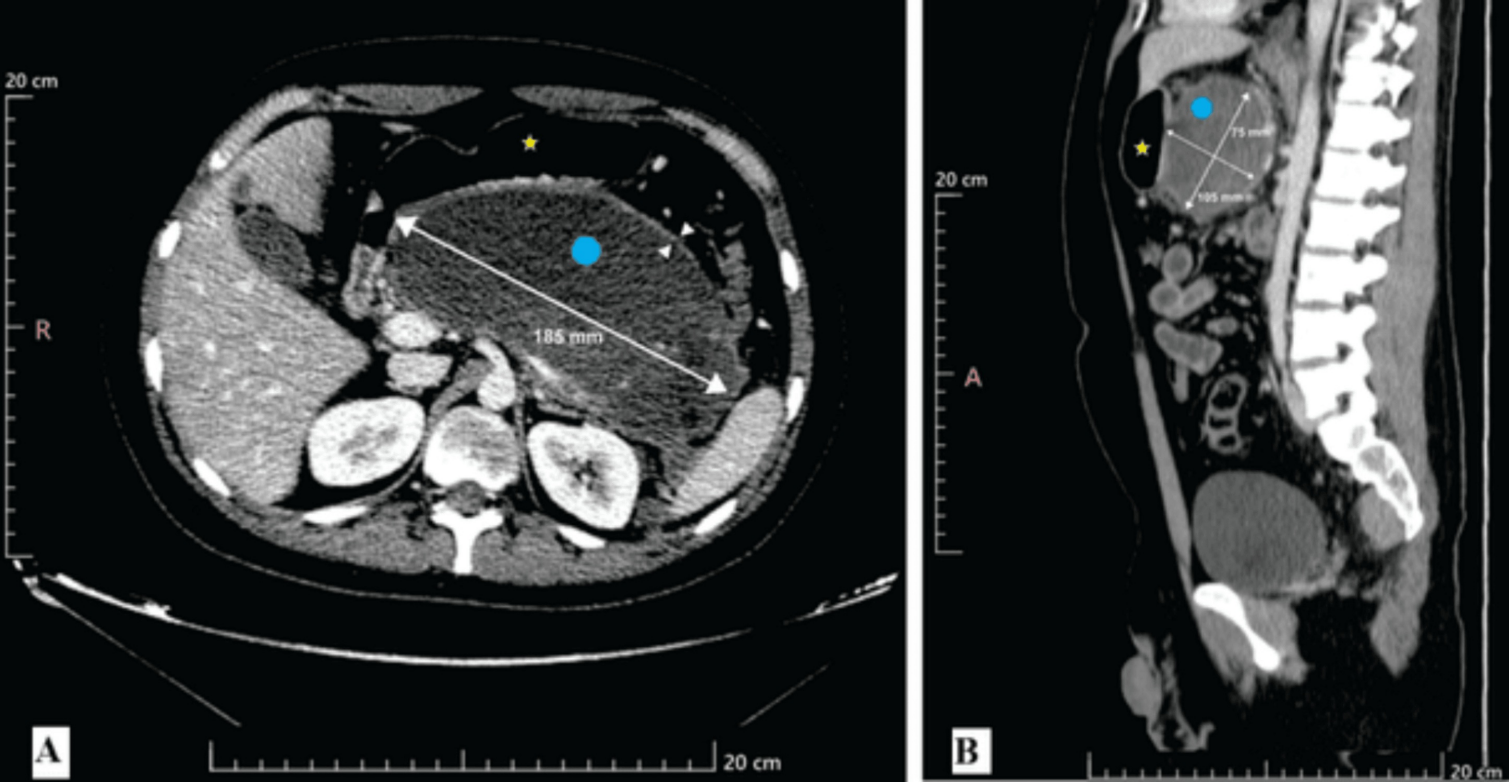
Contrast-enhanced computed tomography of the abdomen The axial view (A) and sagittal view (B) show a large pancreatic pseudocyst (cyan dot) posterior to the stomach (yellow star). The pseudocyst's dimensions are indicated by the measurement lines: 185 mm in the transverse plane, 75 mm in the anteroposterior plane, and 105 mm in the craniocaudal plane
